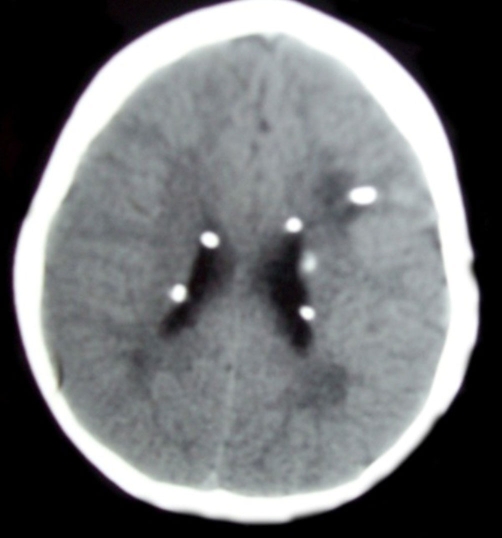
CT scan of the brain showed multiple foci of Subependymal calcification looked like candle-dripping appearances; they were also present in both basal ganglia, both temporal and left parietal regions.
